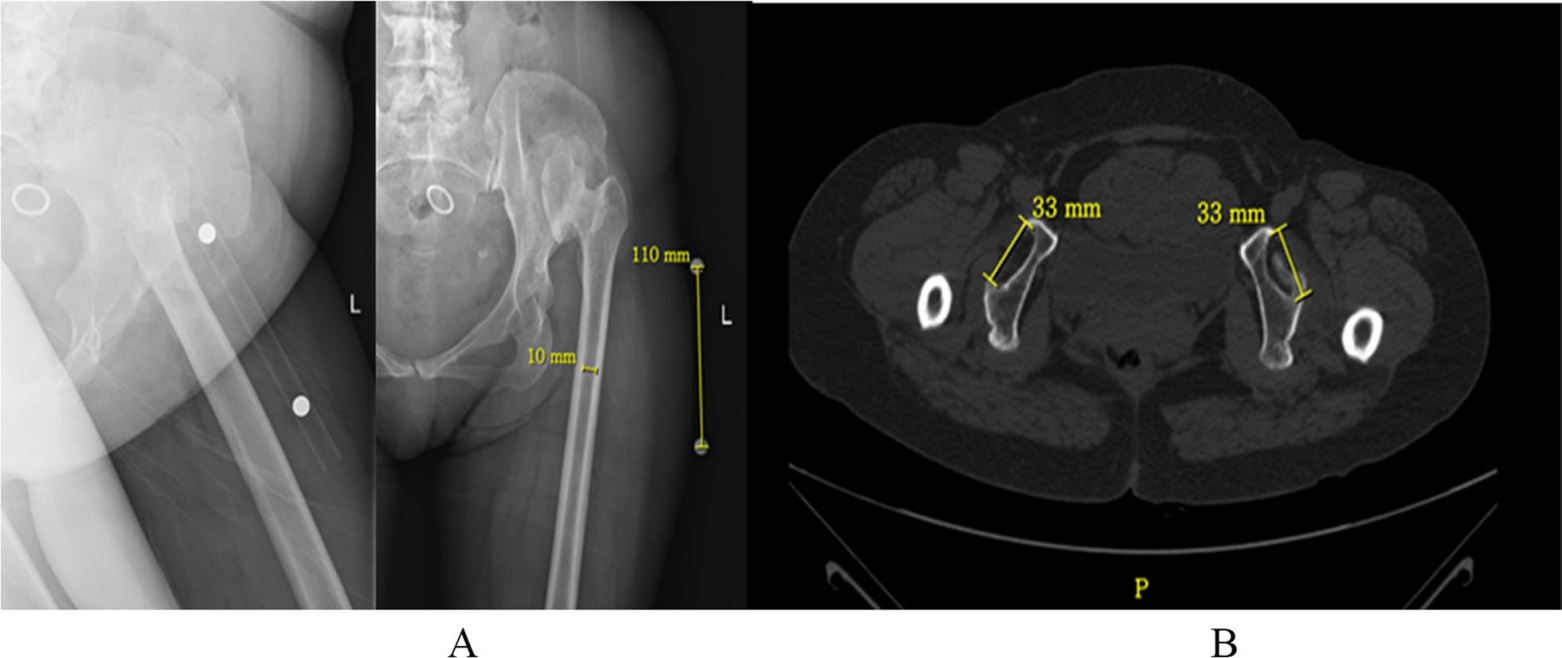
Preoperative measurements were performed using X-ray and CT scans. A We stuck a 100 mm-long marker rod with metal balls on both sides on the outside of the thigh, and took an X-ray of the femur. Measuring the diameter of the medullary cavity at the narrowest part of femoral diameter on X-ray film and calculating the actual diameter of the femoral medullary cavity can avoid the error caused by X-ray magnification and provide the basis for the types of femoral prosthesis that may be used during operation. Photos with marker posts were added. B Preoperative CT scanning of the hip joint was performed to evaluate the position and diameter of the true acetabulum and to guide the selection of acetabular prosthesis size and reconstruction of the true acetabulum
